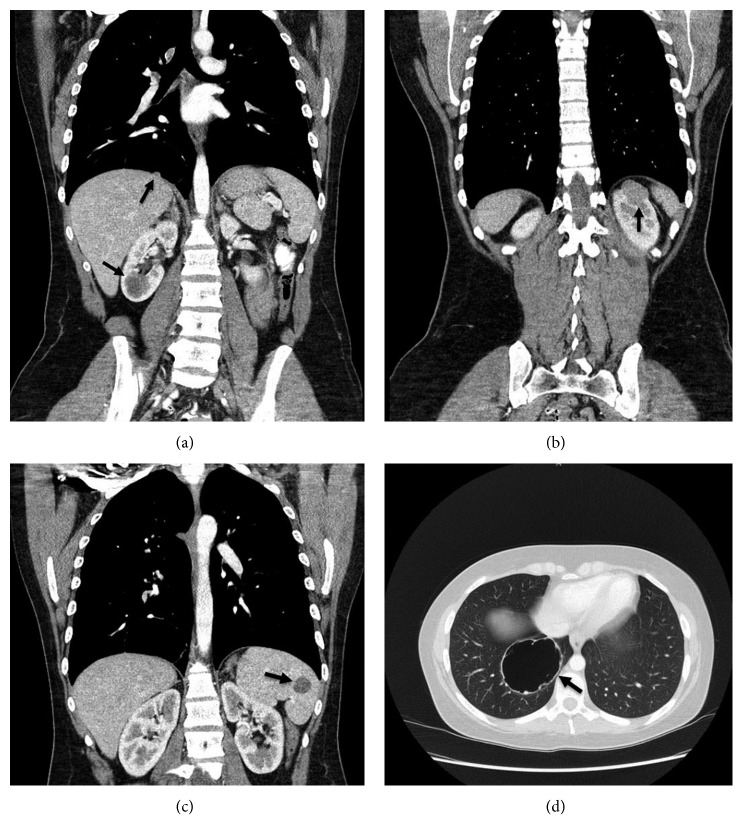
Computed tomography scans of the chest, abdomen, and pelvis demonstrating multiple lesions including a hypodense lesion of the spleen, bilateral kidney lesions, and a large cystic lesion with mural nodularity in the right lower lobe of the lung. The image on the top right demonstrates the lesion that was found to be chromophobe-type renal cell carcinoma in the upper pole of the left kidney, and the image on the bottom right shows the large metastatic adamantinoma lesion in the right lung.
